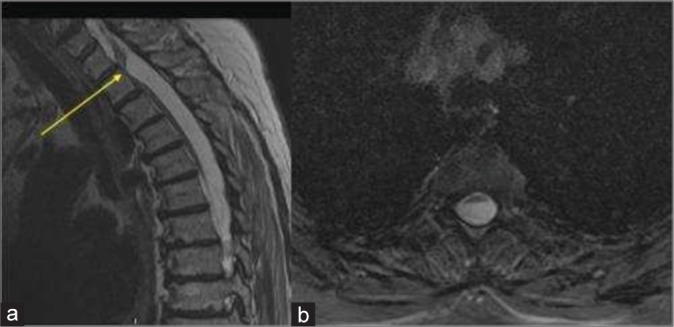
A 76-year-old female who presented with progressive onset difficulty in walking, leg weakness, and gait ataxia. (a) Sagittal T2-weighted and (b) Axial T2 gradient images of the thoracic spine demonstrating anterior cord kink at T2-3 level representing anterior spinal cord herniation (Yellow arrow).
